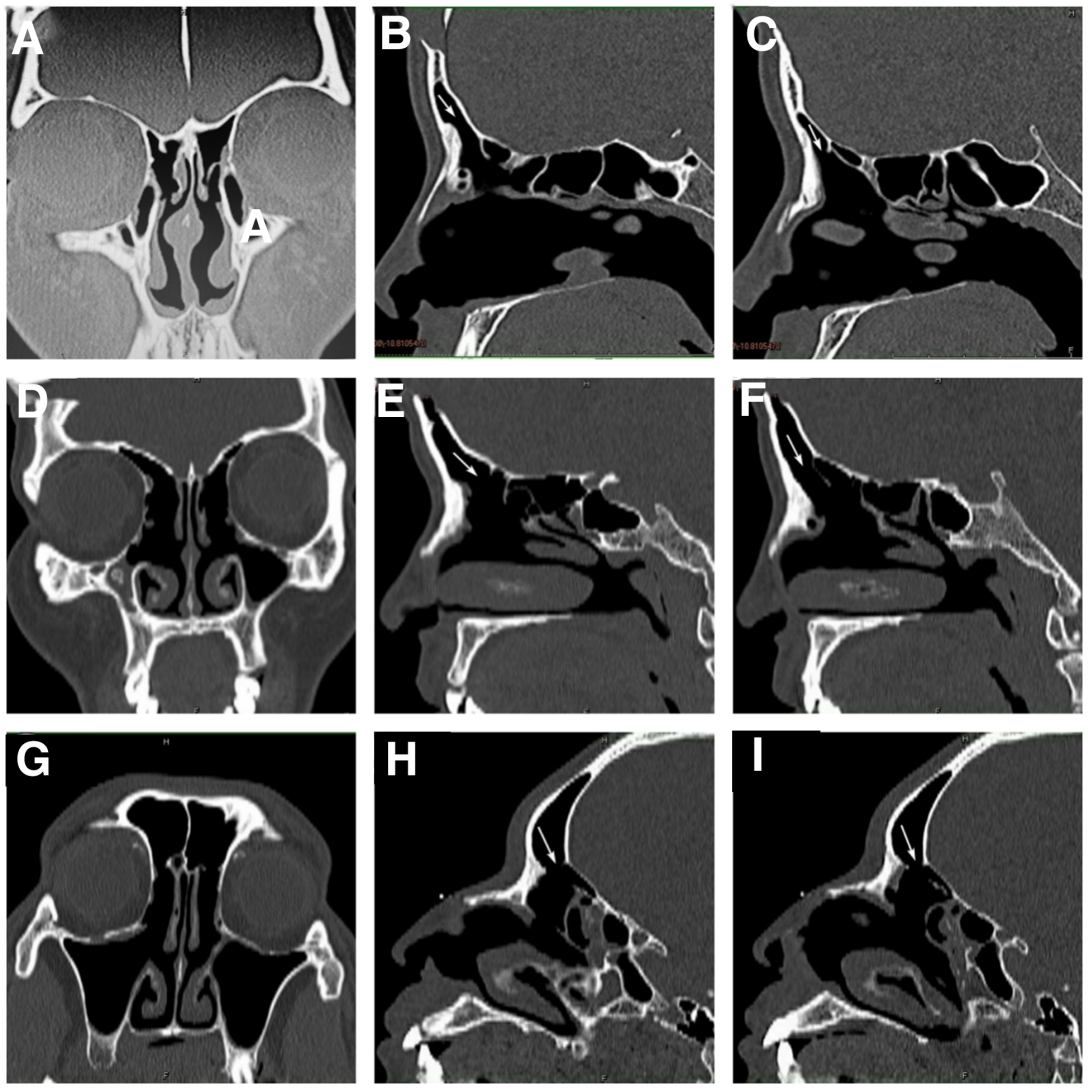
Thin slice CT examination of paranasal sinus in patient 1, 2 and 3 after operation: patient 1 (A–C), patient 2 (D–F), patient 3 (G–I), coronal (A, D, G), sagittal left (B, E, H) and right (C, F, I). The arrow indicates the opening of frontal sinus and the drainage channel of frontal recess.
